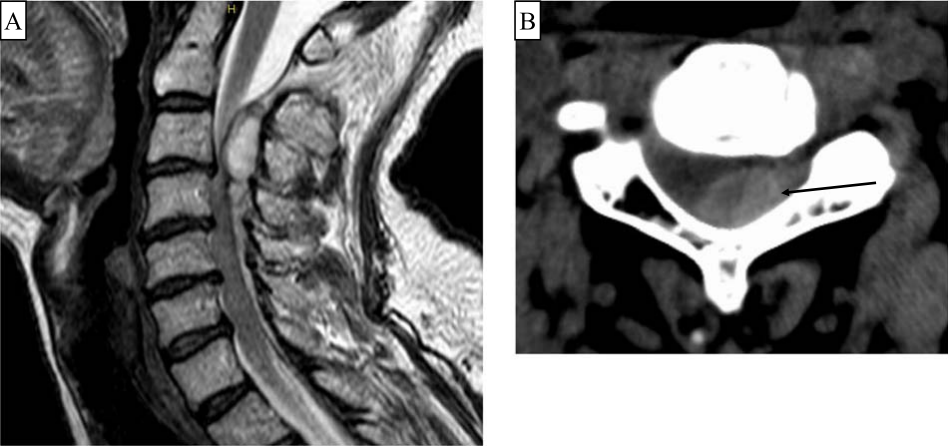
(A) T2-weighted Magnetic resonance imaging (MRI) showing an epidural hematoma at the C2/3 to C4 level. (B) Computed tomography (axial image) showing a hematoma predominantly on the left side at the C3 level (arrow).
